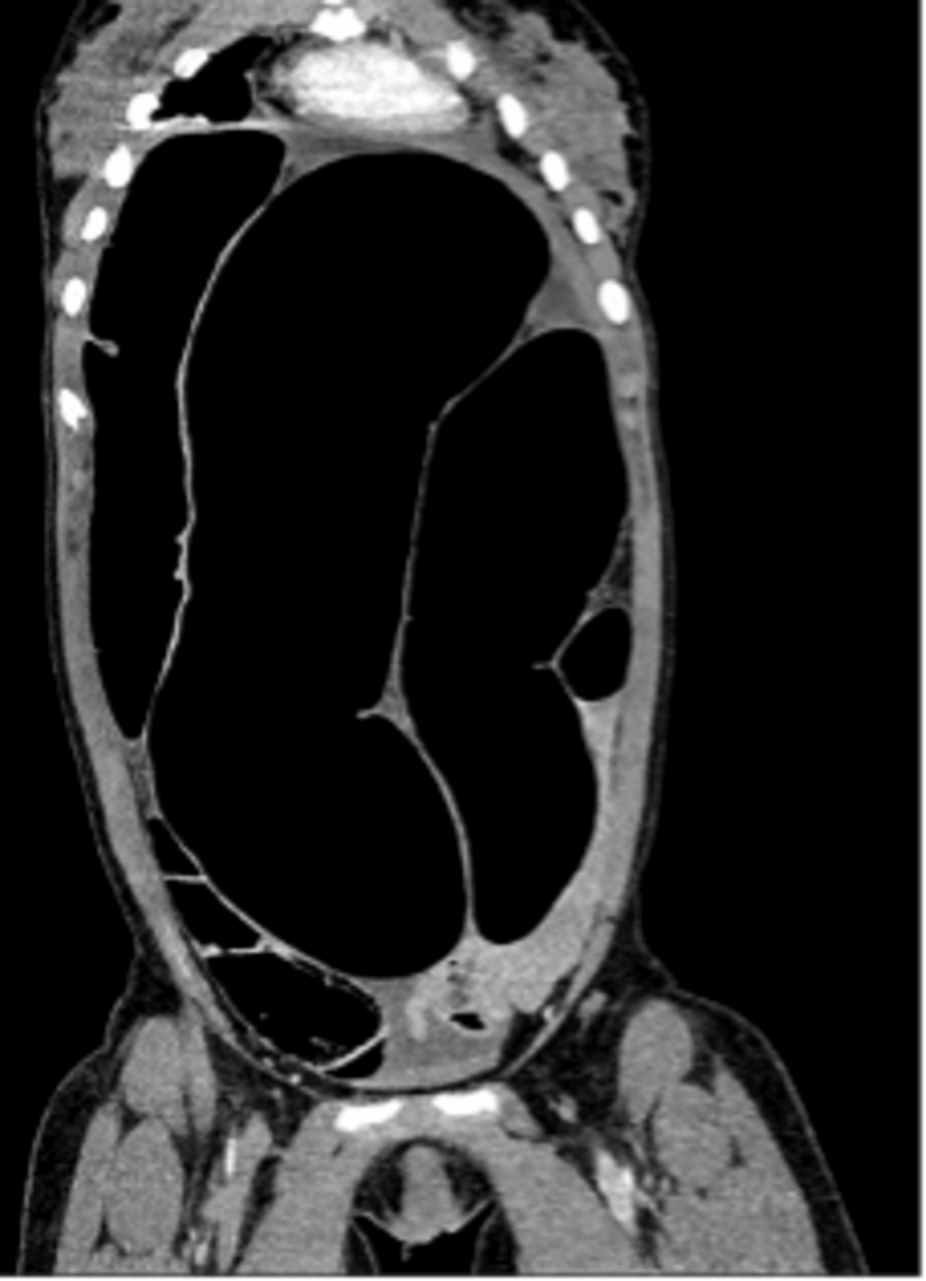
Contrast-enhanced computed tomography of the abdomen and pelvis (CT AP) showing the dilated loop of the large bowel
